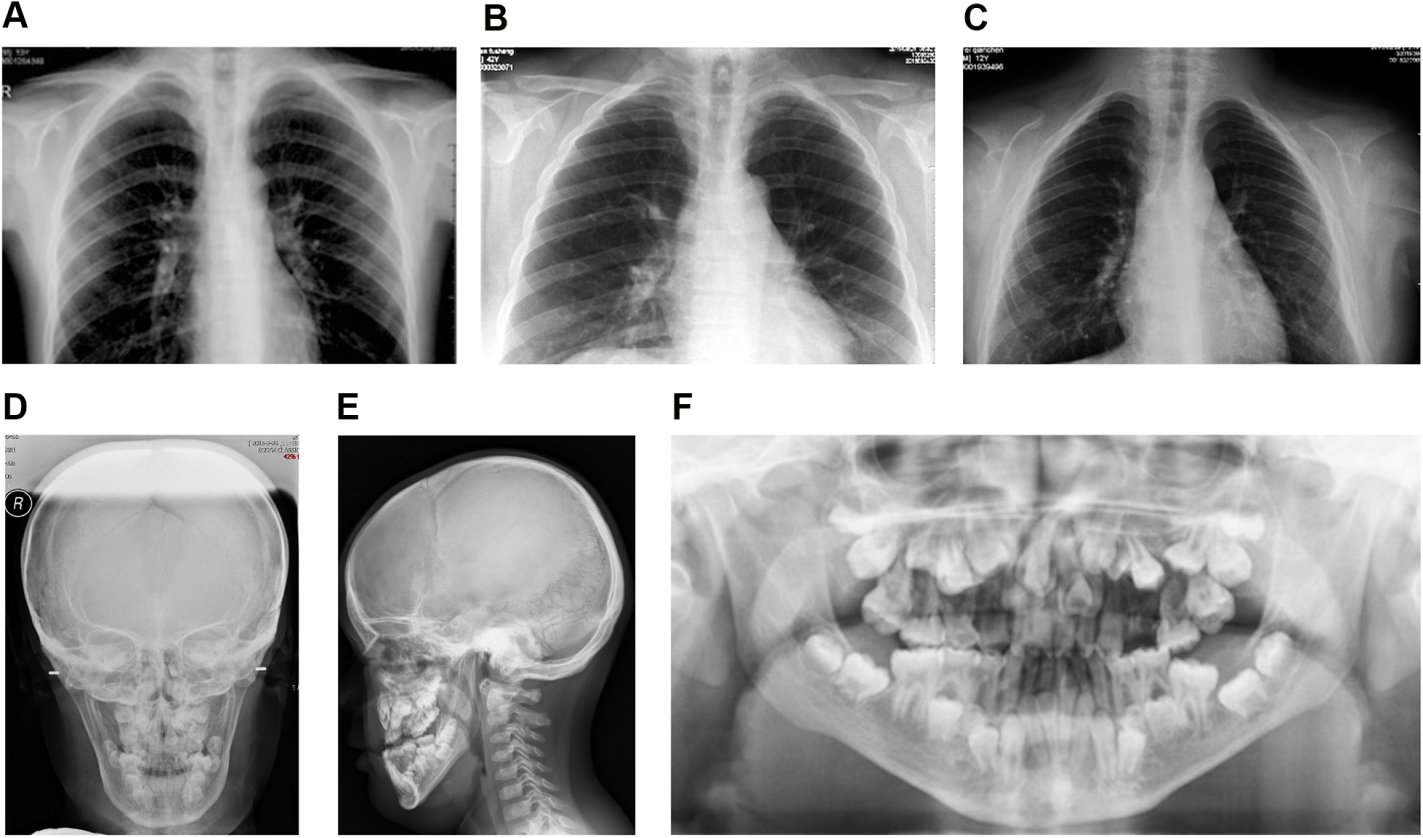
Radiographic manifestations of Patient 2, Patient 3 and Patient 4. (A-C) Chest radiographs of Patient 2 (A), Patient 3 (B), and patient 4 (C). (D-F) Clinical radiographs of Patient 4. Anteroposterior (D), lateral (E), and panoramic radiographs (F) of Patient 4.
